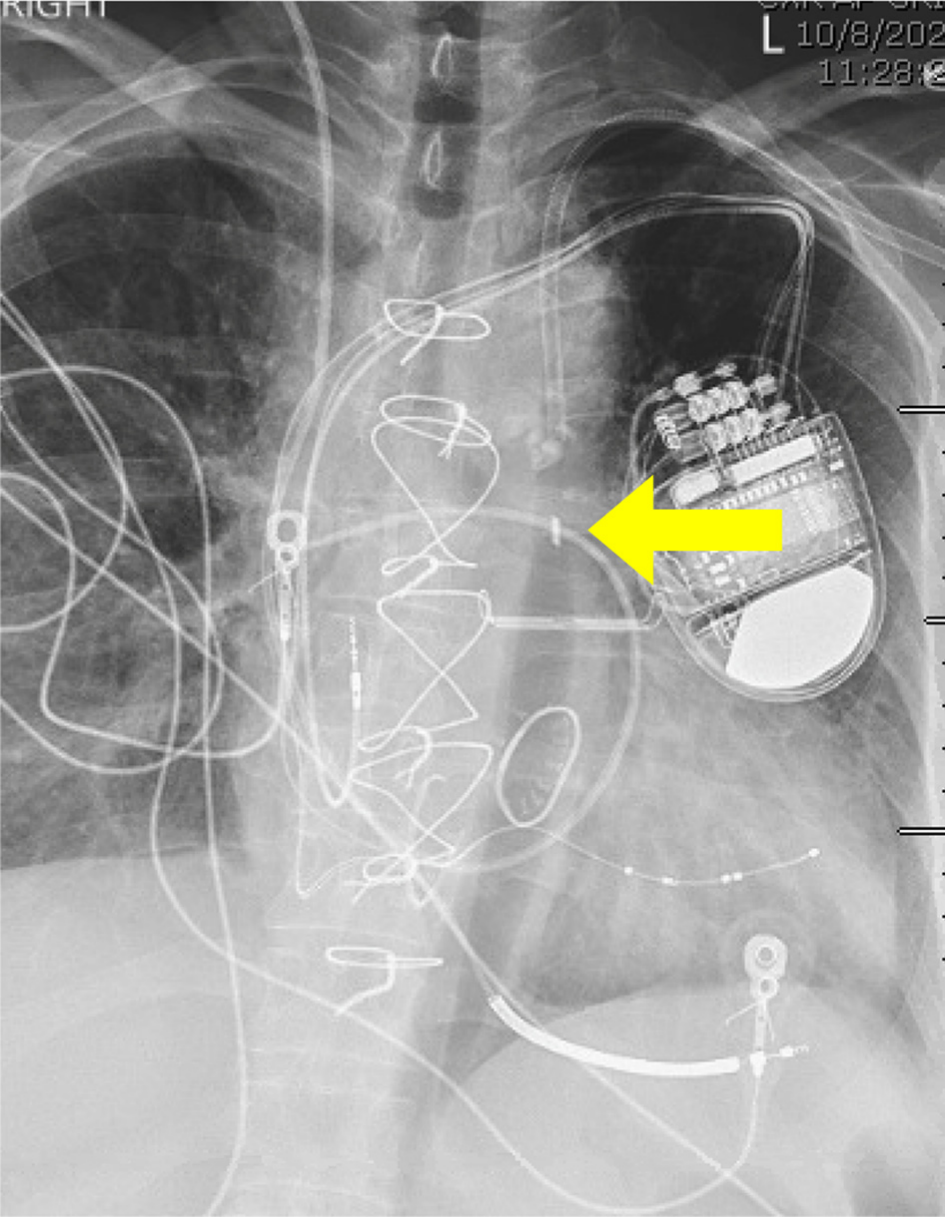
Chest x-ray after axillary intra-aortic balloon pump (IABP) placement. The cranial IABP radiopaque marker is seen in the descending aorta at the level of the left mainstem bronchus (*yellow arrow*).
